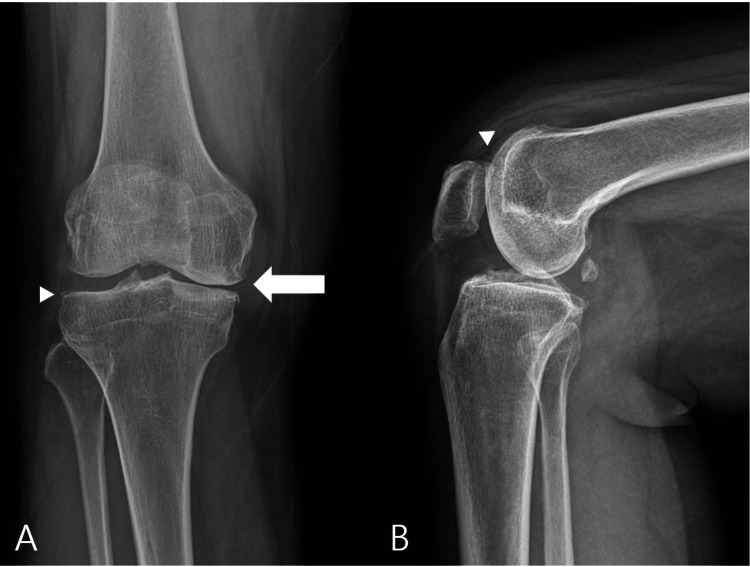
Baseline radiographic findings of the right knee from the initial visit A: The anteroposterior radiograph demonstrates medial compartment joint-space narrowing, marginal osteophyte formation, and degenerative changes consistent with Kellgren-Lawrence grade III osteoarthritis. The white arrow indicates medial compartment joint-space narrowing, and the white arrowhead indicates marginal osteophyte formation. B: The lateral radiograph demonstrates degenerative osteoarthritic changes, including patellofemoral osteophyte formation, indicated by the white arrowhead.
